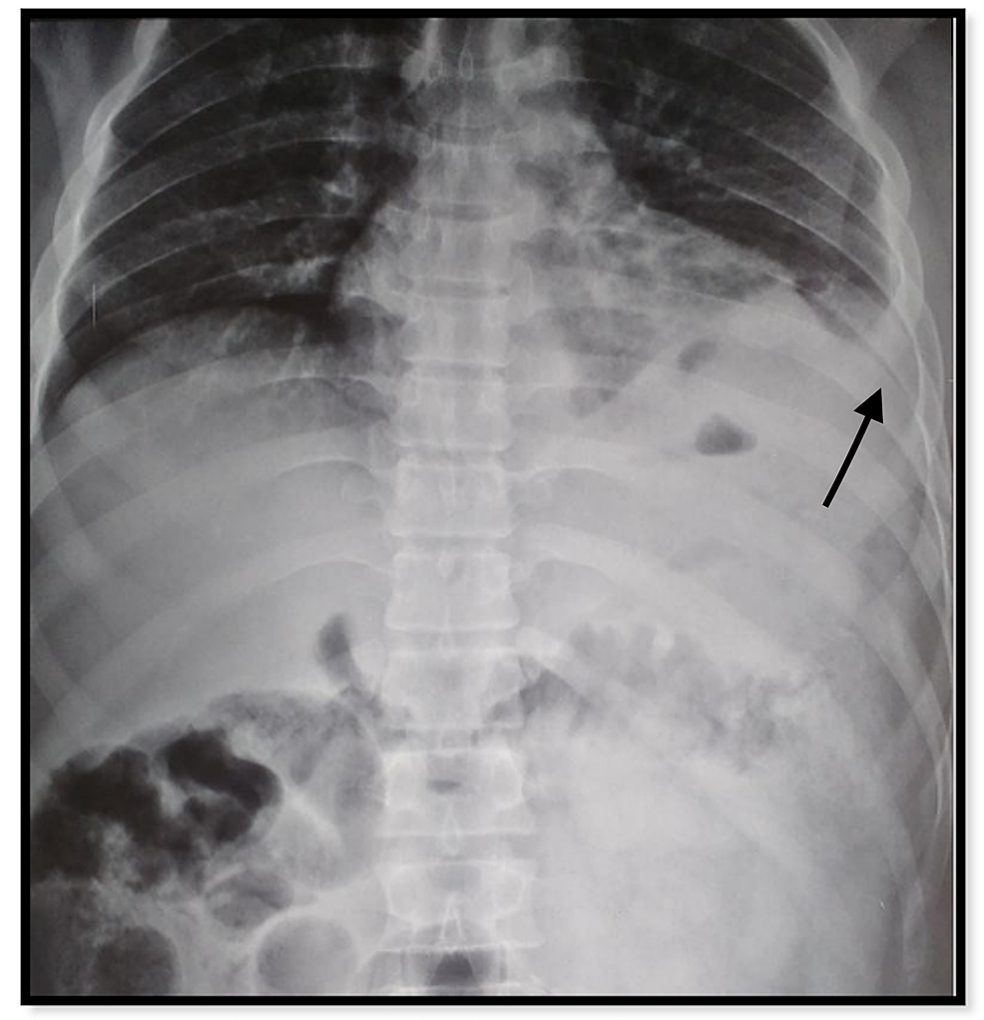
The initial X-ray at the time of injury The injury was misinterpreted as left hemothorax (black arrow)
